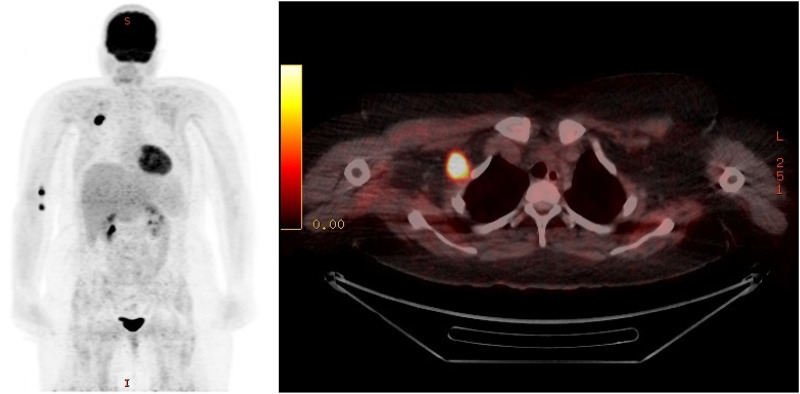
^18^F­FDG-PET/CT image showing a high glucose uptake in the right axillary lymph node, while no other hypermetabolic lesions were detectable (permission was granted by the patient).
